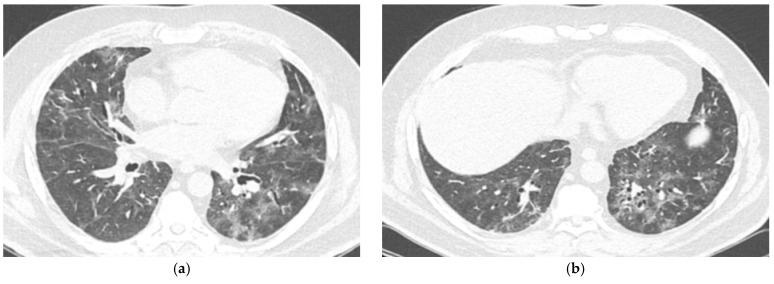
Rapidly advancing pulmonary involvement on chest CT imaging in an 45 year old patient. (a) Chest CT scan showing bilateral diffuse ground-glass opacities in the lower lobes. (b) Chest CT scan demonstrating progression with extensive ground-glass opacities and interlobular septal thickening, involving approximately 50% of the pulmonary parenchyma.
